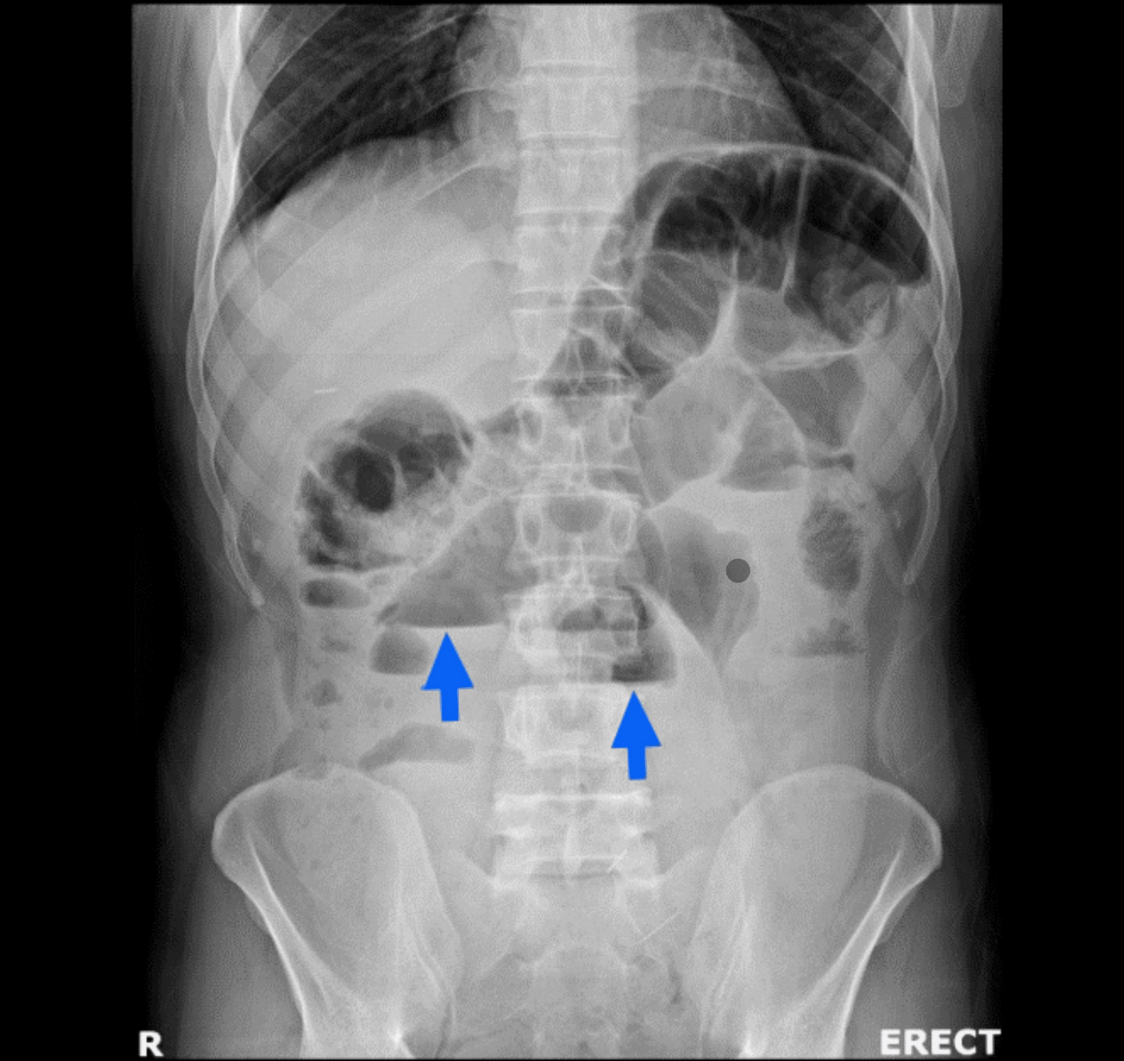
Erect plain abdominal radiograph demonstrating centrally located dilated small bowel loops with multiple air-fluid levels (blue arrows), suggestive of small bowel obstruction.
